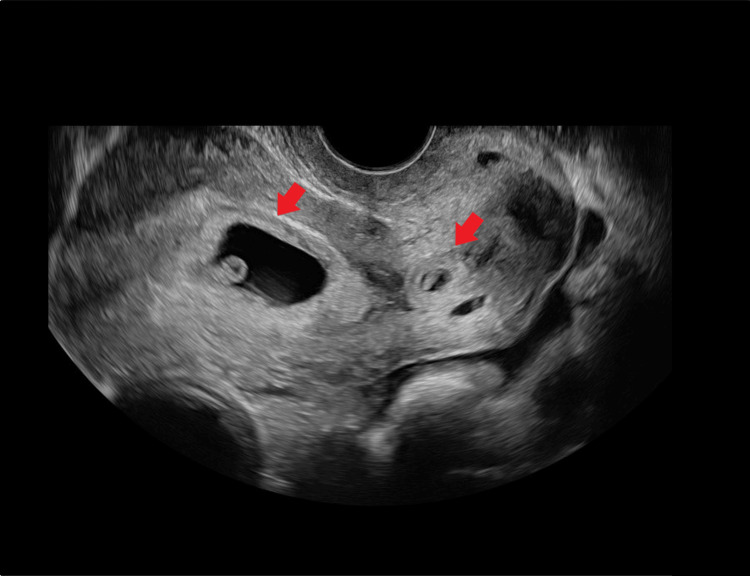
Transvaginal ultrasonography showed an intrauterine and a cervical pregnancy simultaneously at six weeks and four days of gestation Arrows are showing the two gestational sacs.
